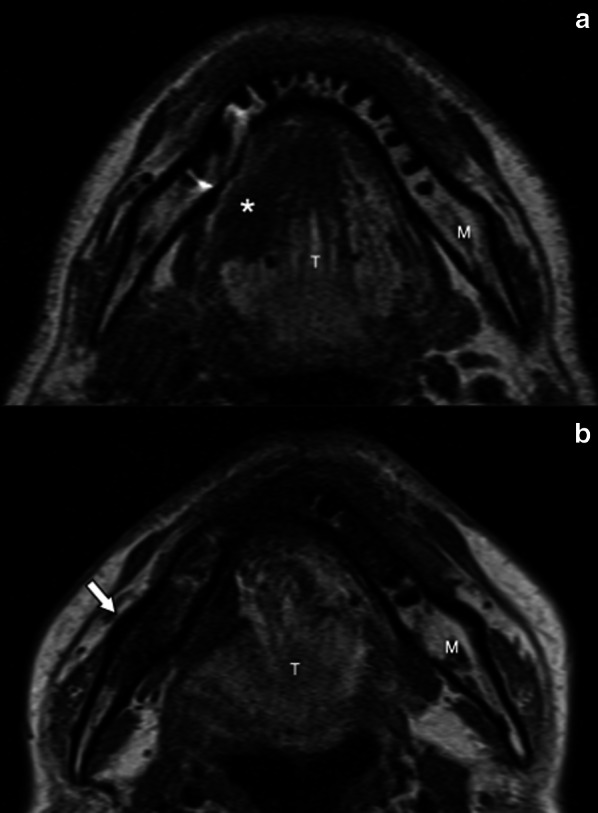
Osseous sclerosis of the right ramus of the mandible (arrow) in a 44-year-old man 5 years after right hemiglossopelvectomy followed by adjuvant ChT/RT for a carcinoma of the right border of the tongue. Pretreatment axial T1-weighted (a) MR image shows a lesion of the right border of the tongue (asterisk) and normal signal intensity of the right ramus of the mandible. Post-treatment axial T1-weighted (b) MR image shows the surgery outcome and diffuse hypointense signal of the spongiosa of the right ramus of the mandible (arrow). M, mandible; T, tongue
